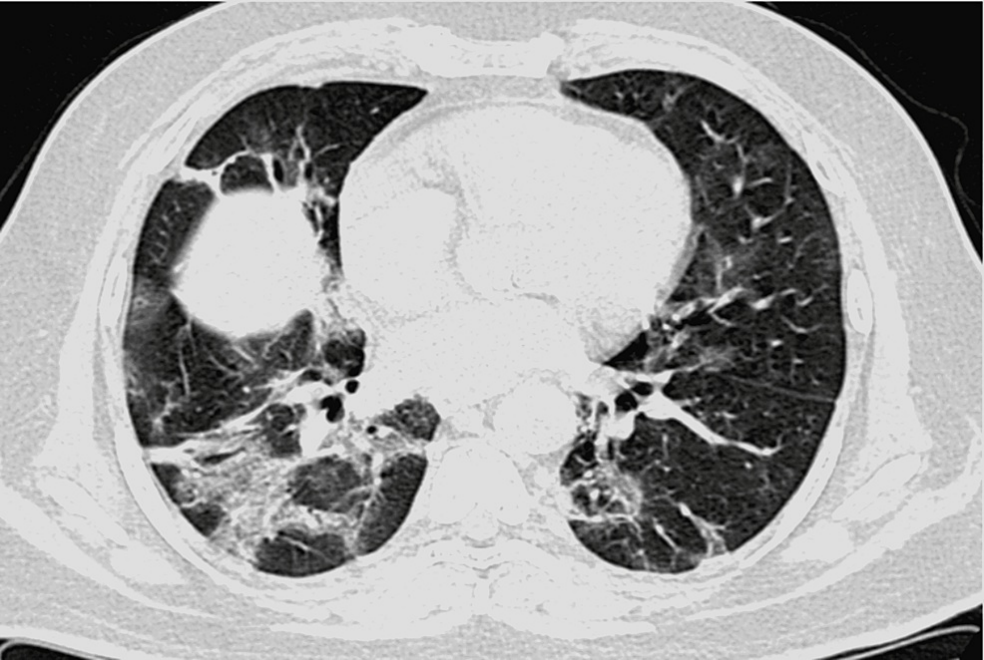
Axial CT section of the chest showing consolidation in a patient with COVID-19 pneumonia
